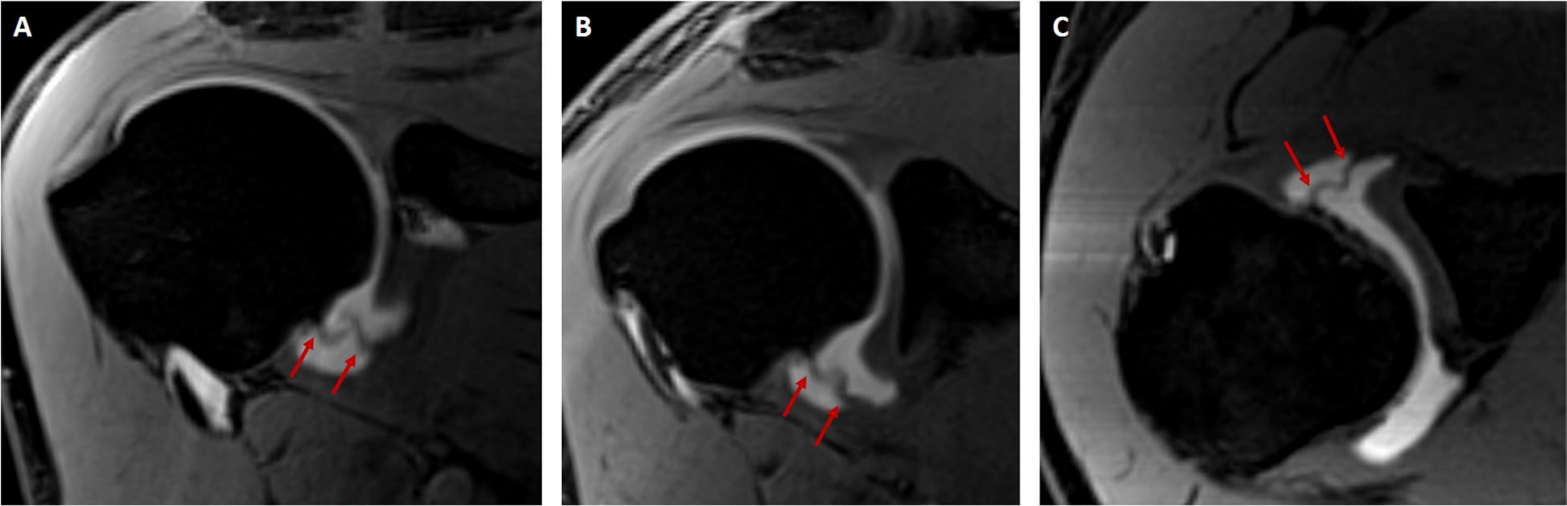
Consecutive oblique coronal A, B and axial C thin-slice VIBE T1-weighted MR arthrograms show SpiGHL (red arrows) without conjugation with MGHL
